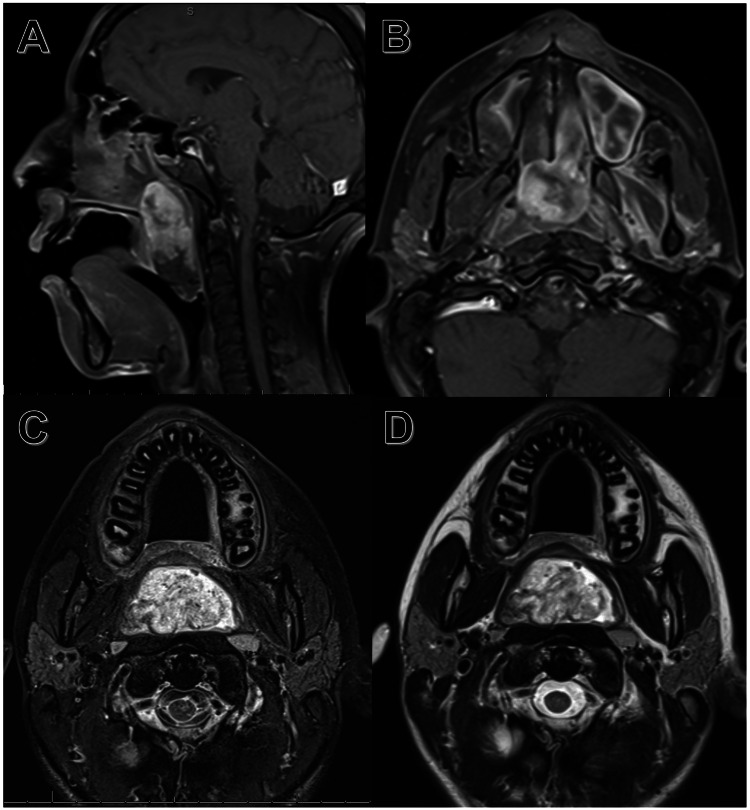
MRI face/paranasal sinuses with and without contrast (A) T1-weighted MRI image with contrast showing the nasopharyngeal polypoid mass. (B) T1-weighted MRI image with contrast showing extension into the left masticator space. (C) Short tau inversion recovery (STIR) MRI image without contrast showing a hyperintense mass. (D) T2-weighted MRI image without contrast showing a hyperintense mass.
